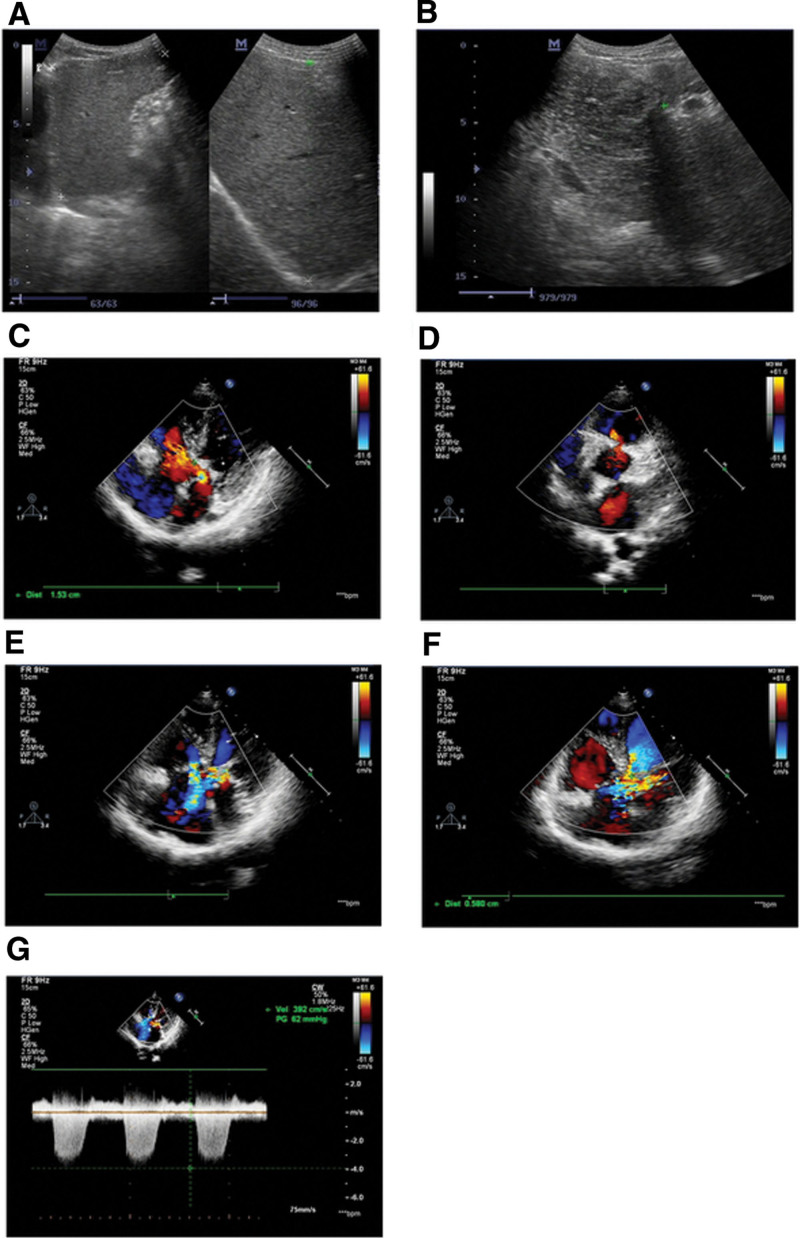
Proband ultrasonogram and echocardiography results. Ultrasonogram images show enlarged (A) liver and (B) spleen. (C–G) Echocardiography revealing endocardial cushion defect, including (C) atrial septal defect, (D) ventricular septal defect, (E) root interruption of anterior mitral valve, (F) echo interruption of tricuspid septal valve, and (G) pulmonary hypertension.
